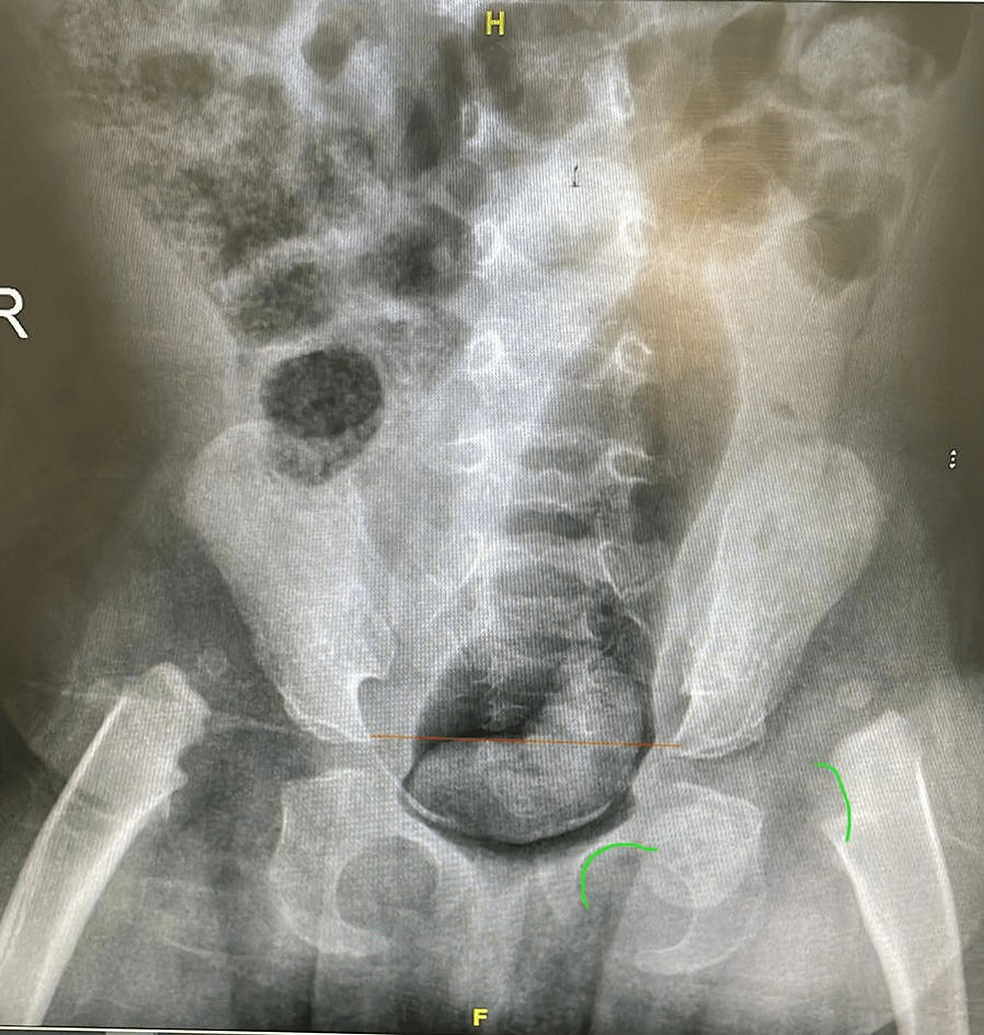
Shenton's line (green lines). The acetabular index (red line) is the main parameter to control acetabular development during the first years of age. The X-ray was obtained from one of the patients in the clinic.
